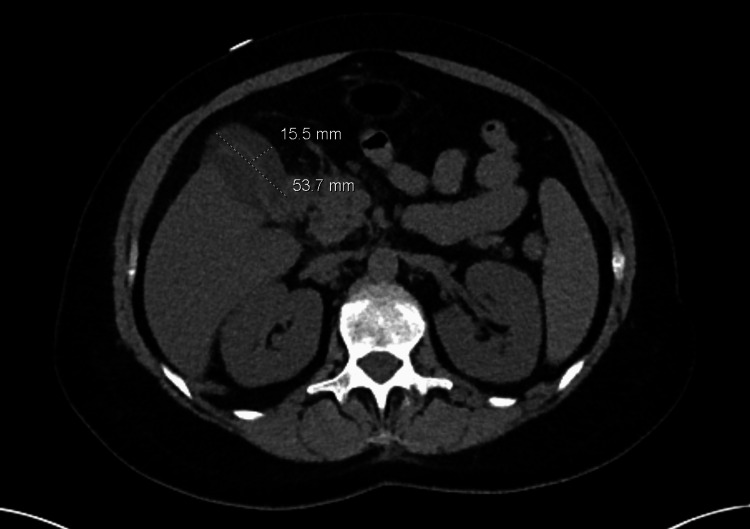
CT A/P showing a fluid collection adjacent to the gallbladder measuring up to 1.6 x 5.4 cm. CT: computed tomography; A/P: anterior-posterior
